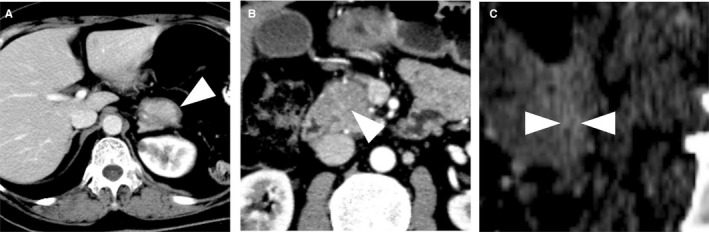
A, A capsule‐like rim is shown as a hypo‐attenuating rim in the surrounding adipose tissue in the axial enhanced computed tomography (CT) images (arrow). B, A “duct‐penetrating sign” can be detected within the lesion presenting as the lumen of the main pancreatic duct (MPD) in the axial CT images (arrow). C, An “enhanced duct sign” is observed in the coronal reconstructed enhanced CT image, showing an enhancement of the MPD wall in the lesion (arrow)
